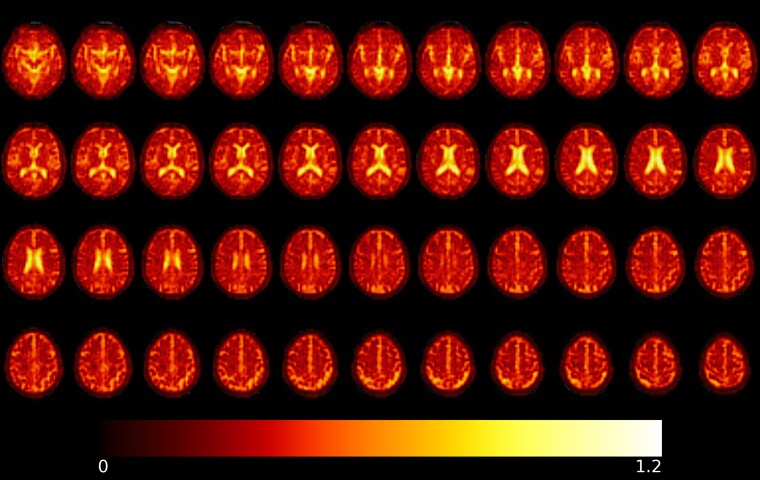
Prenormalized sodium image. The sodium image is B0 and B1 inhomogeneity corrected overlayed on a T1-weighted image for a 72 year-old male, apoe4 non-carrier, ADASCog11 score = 6 (range of sodium values: 0–1.2 with colour bar).
